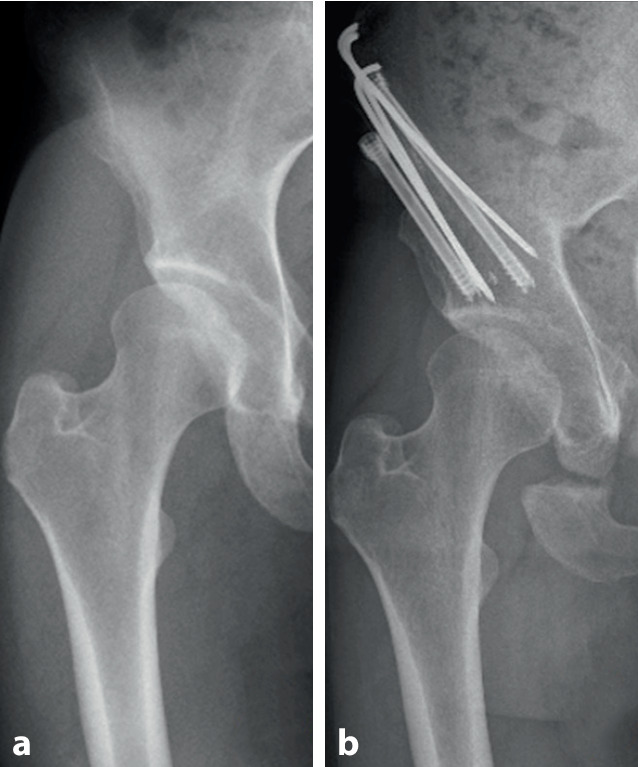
AP-radiographs of a 33-year-old female patient preoperatively (a) and 1 year postoperatively (b)
